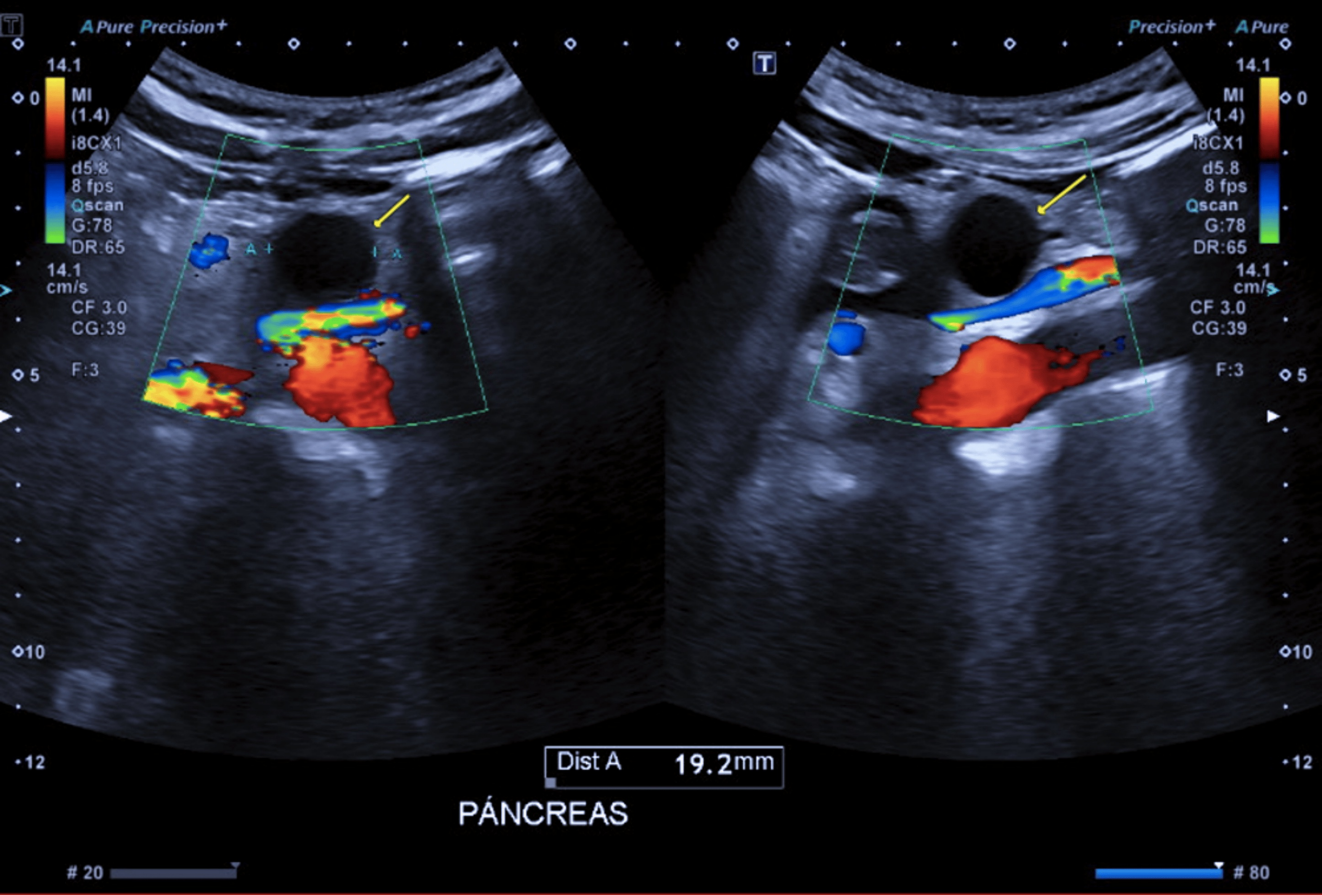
Abdominal ultrasound In the body of the pancreas, a cystic lesion with a nonaggressive appearance is identified, measuring 22 mm in its largest diameter. It does not cause retrograde dilation of the main pancreatic duct. Further evaluation with magnetic resonance imaging is recommended
